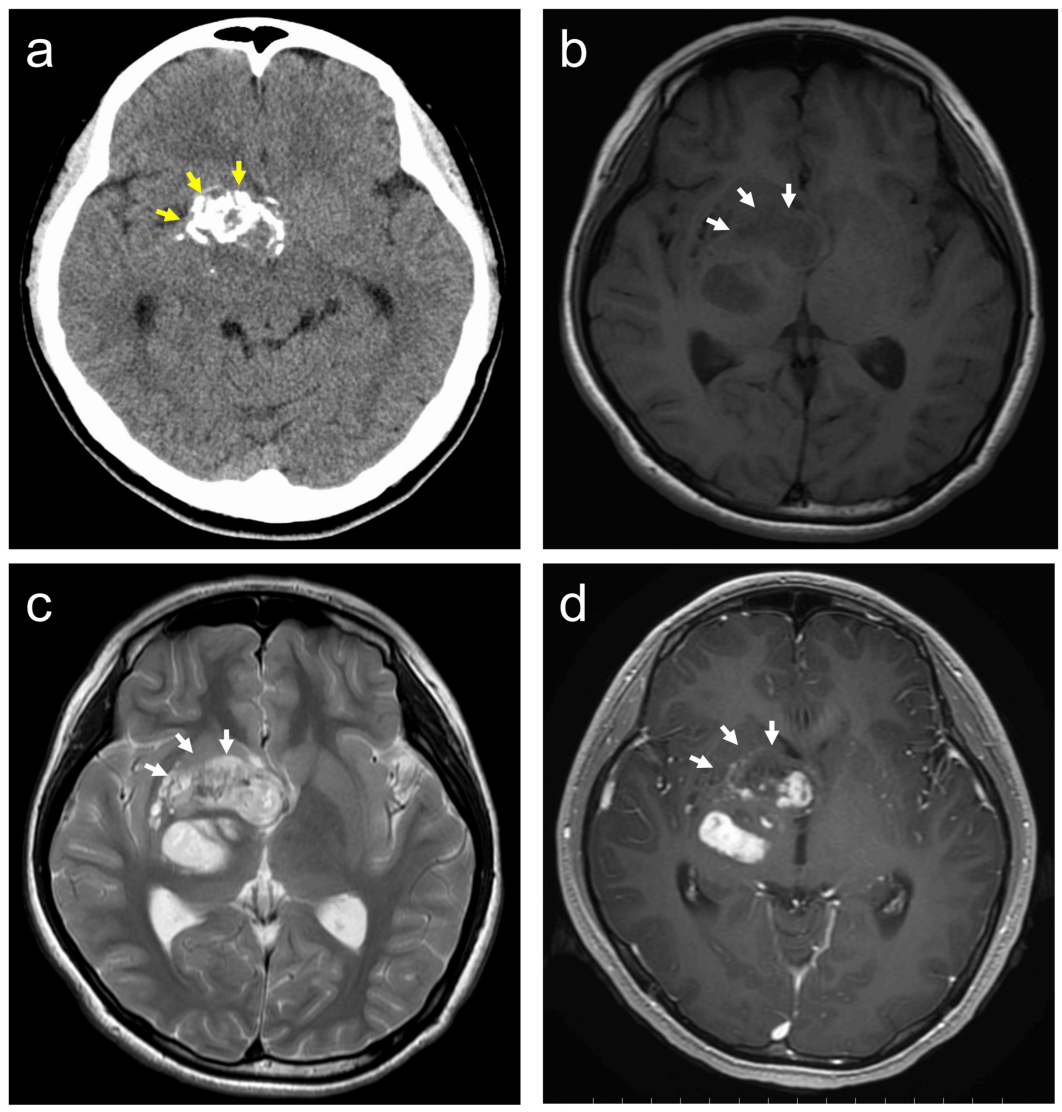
Initial radiological findings. (a) Intratumoral calcification (yellow arrows) on computed tomography. (b,c) Magnetic resonance imaging (MRI) revealed hypointensity on T1-weighted images (WI) and heterogeneous hyperintensity on T2-WI of the tumor (arrows). (d) Contrast-enhanced T1-WI showing heterogeneous enhancement within the solid component of the tumor (arrows).
